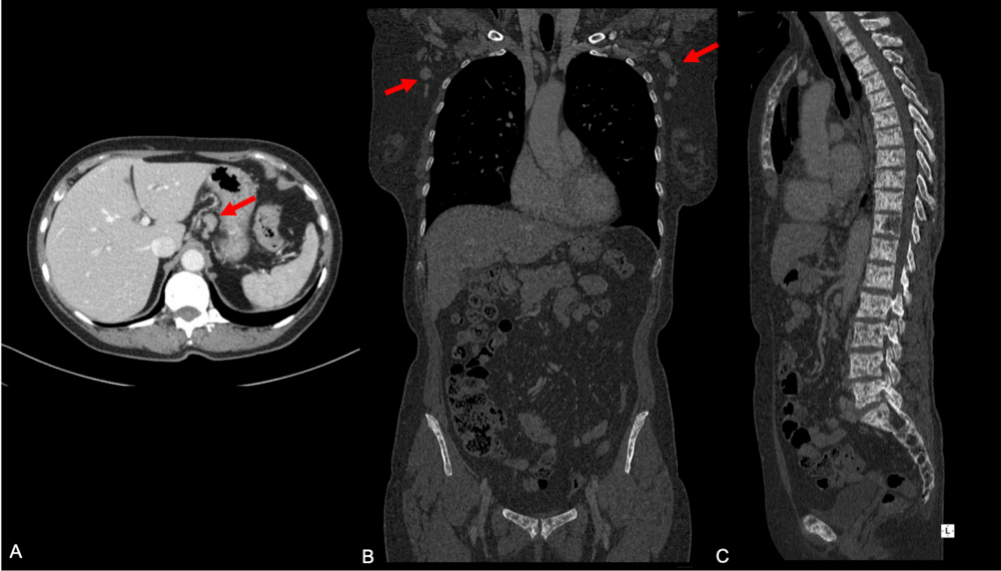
Contrast-enhanced body CT. (A) axial, (B) coronal and (C) sagittal plane showing suspicious node in the abdomen and in the axilla (A, B). It also shows (B, C) diffuse bone-involvement, with mixed both osteolytic and osteoblastic features, which interested almost every skeletal structures of the body (vertebral bodies of the entire column, costal skeleton, sternum, proximal third of both humeri, scapulae, clavicles, pelvis and femurs).
